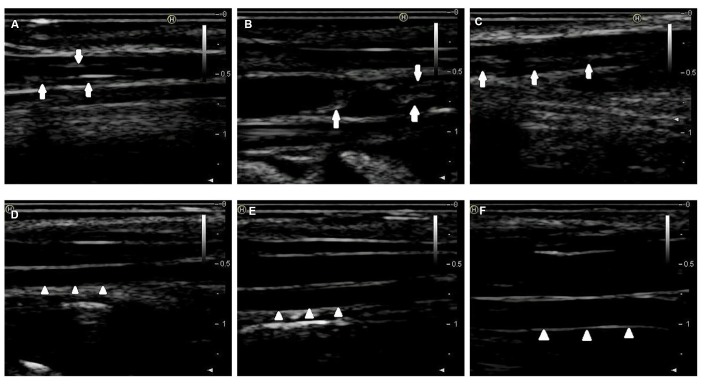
2D-ultrasound images of rabbit carotid arteries at the 12^th^ week. (A–C) 2D-ultrasound images reveal that obvious atherosclerotic plaques formed on rabbit carotid arterial intima as the arrows show. (D–F) 2D-ultrasound images demonstrate that carotid arteries intima of rabbits treated with normal chow still maintain smoothness as the arrows show.
